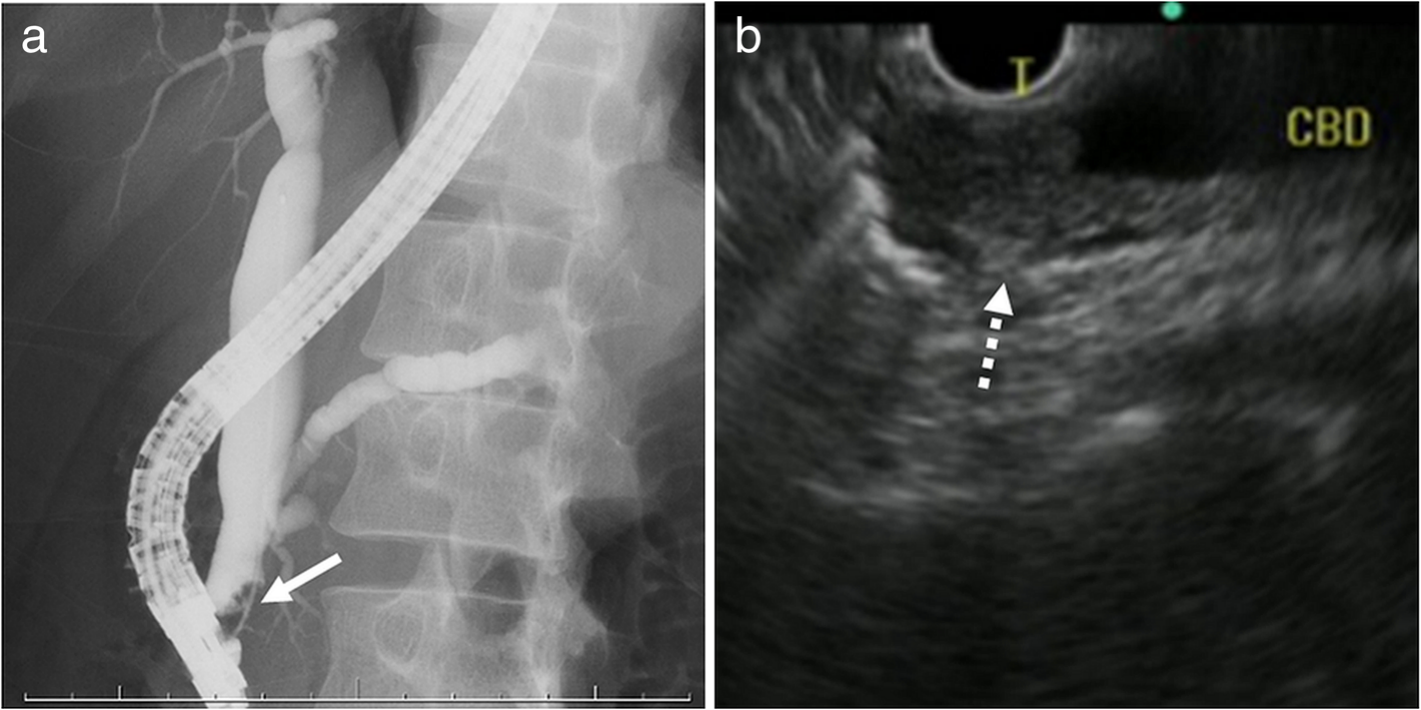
Endoscopic retrograde cholangiopancreatography detected findings similar to those of the MRI and MRCP (*arrow*) (a), and IDUS detected a torose lesion (*dotted arrow*) at the lower CBD (b)
